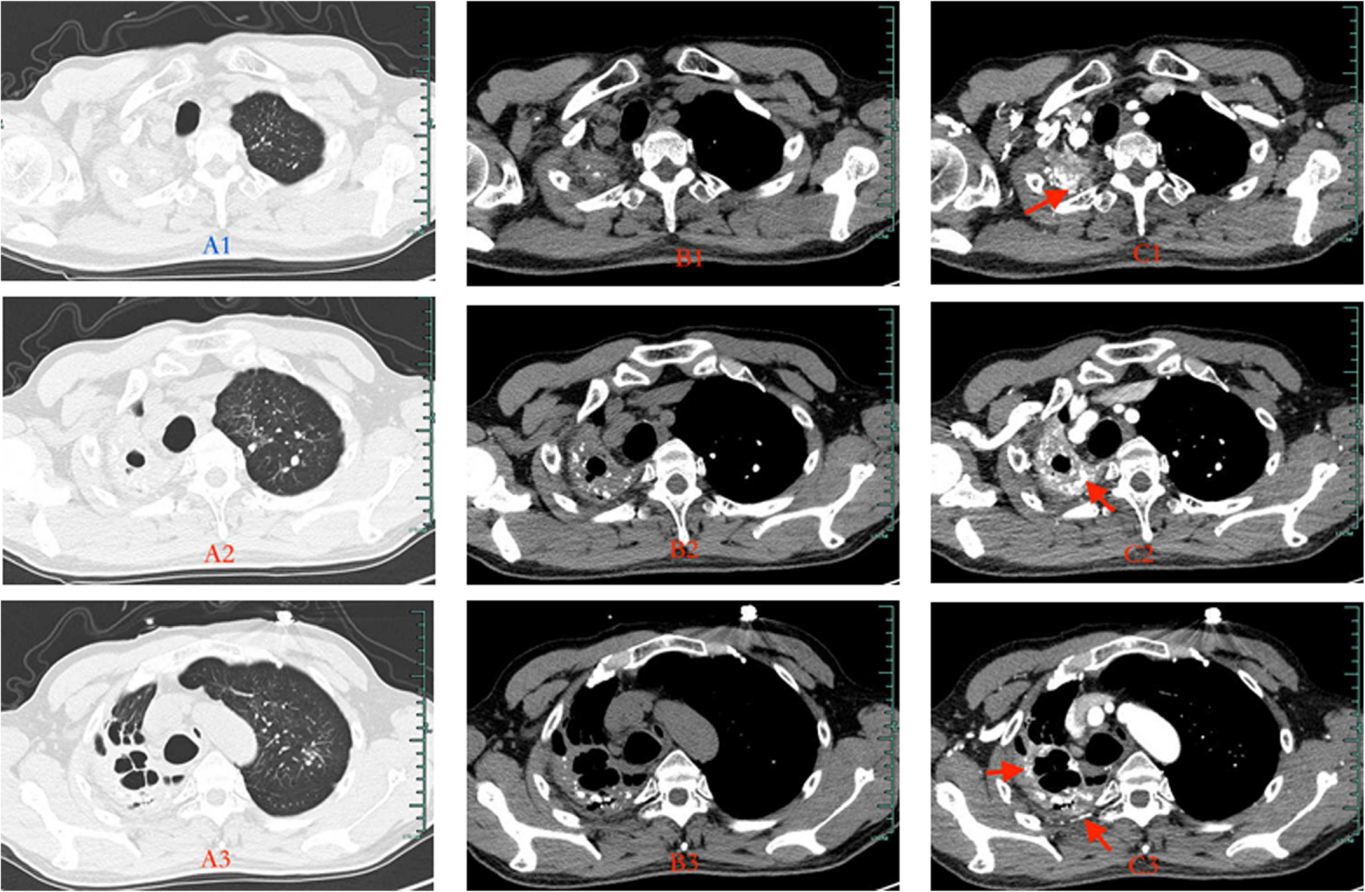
MSCT images taken before regional arterial embolization (male, 58 years old, repeated episodes of hemoptysis for more than 2 years; after two intervention treatments, hemoptysis was still observed, the largest amount of hemoptysis was 500 mL): vascular enhanced CT showed an increased supply of blood at the pleura
